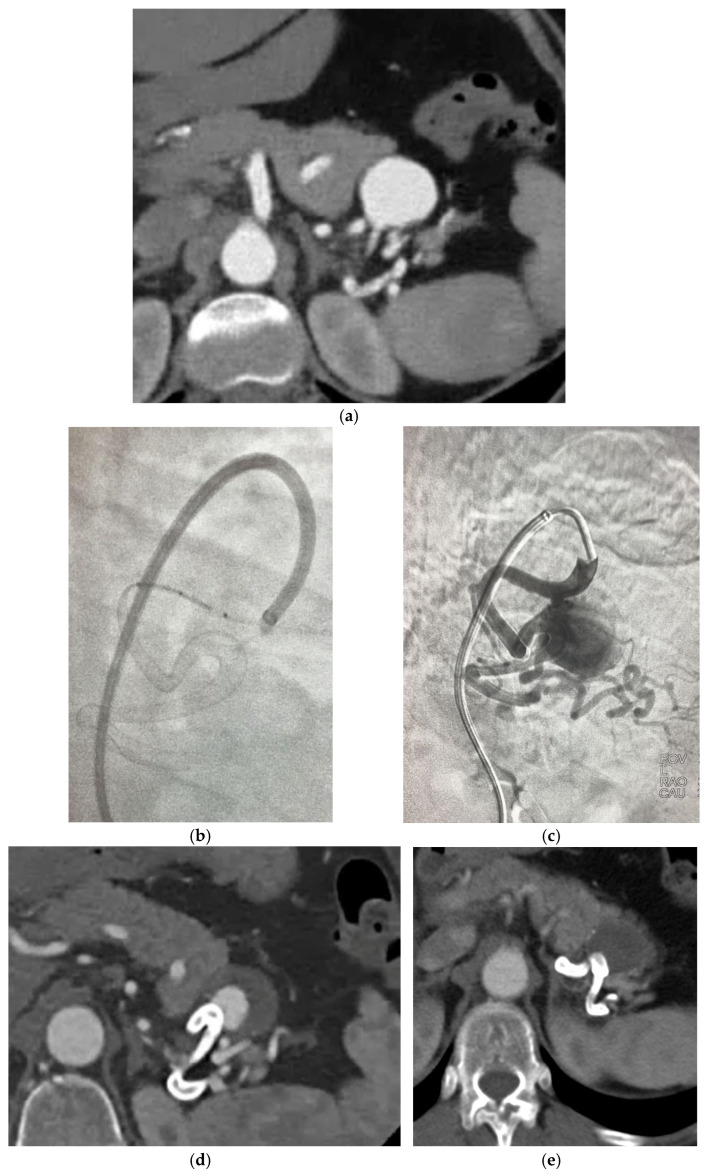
(a) Angio—CT shows 4-cm splenic distal artery aneurysm with three efferent vessels. (b,c) DSA showing the placement of a FD Derivo 6 × 5 cm. (d,e) Angio CT of 1 and 6 months FU with partial and total thrombosis of the sac. Patency of efferent vessels.
